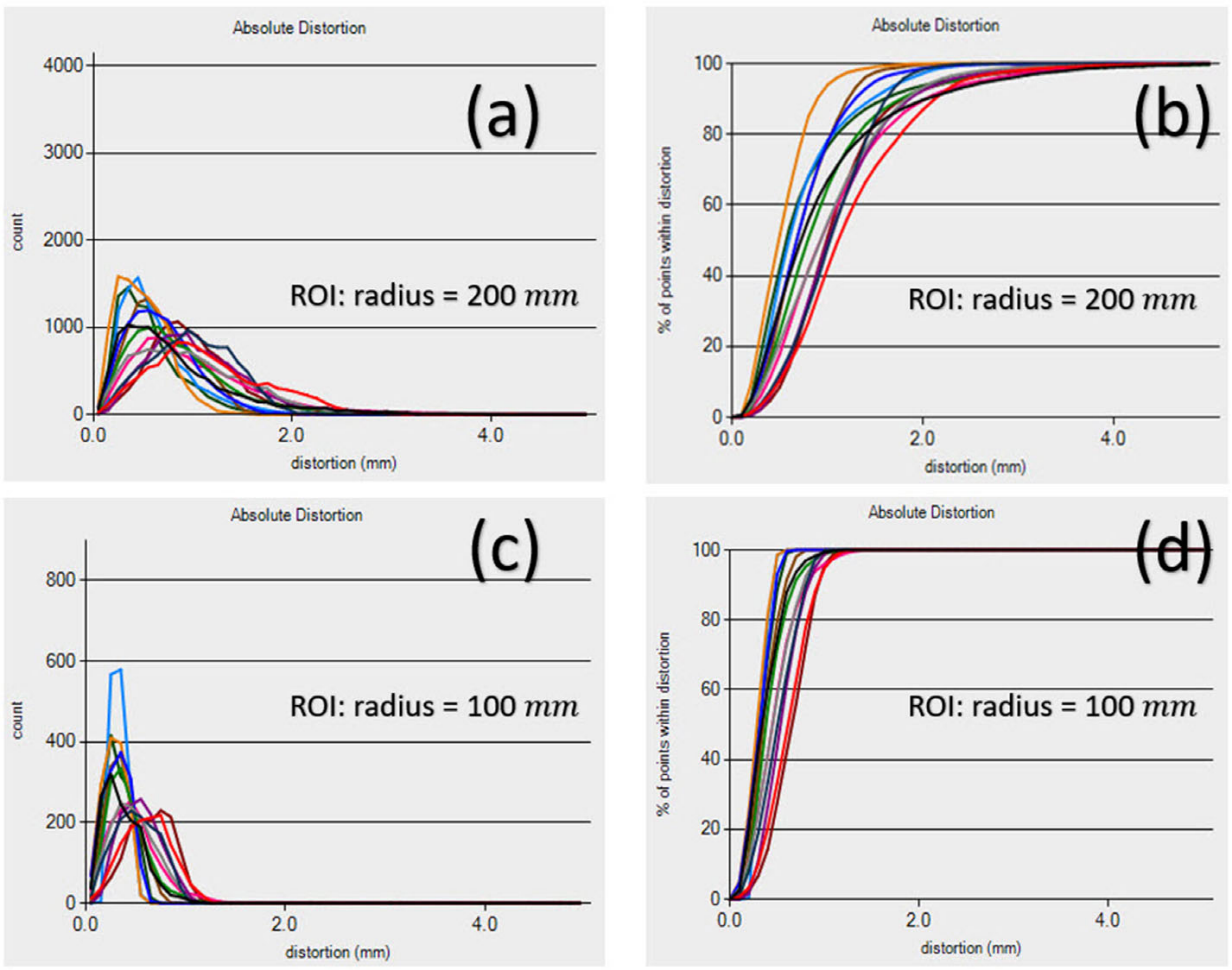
(a) The histogram (b) percentage distribution of control points versus image distortion absolute magnitude for the region of interest (ROI) within the radius of 200 mm for 13 MRI scanners, parts (c) and (d) are those for the ROI within the radius of 100 mm for 13 MRI scanners.
